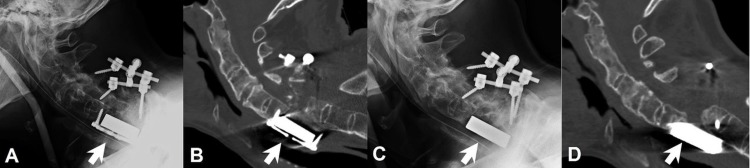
Dislodgement of the plate and cage A: Lateral radiograph taken after the initial surgery. B: Cervical computed tomography (CT) scan at three weeks postoperation, demonstrated the dislodgement of the plate and cage in the prevertebral space. C: Lateral radiograph taken at two months after the second operation, which could only remove the dislodged screws and plate and left the dislocated metallic cage. D: Cervical CT scan taken at four months after the second operation demonstrated that the dislodged cage seemed stabilized after the reoperation.
